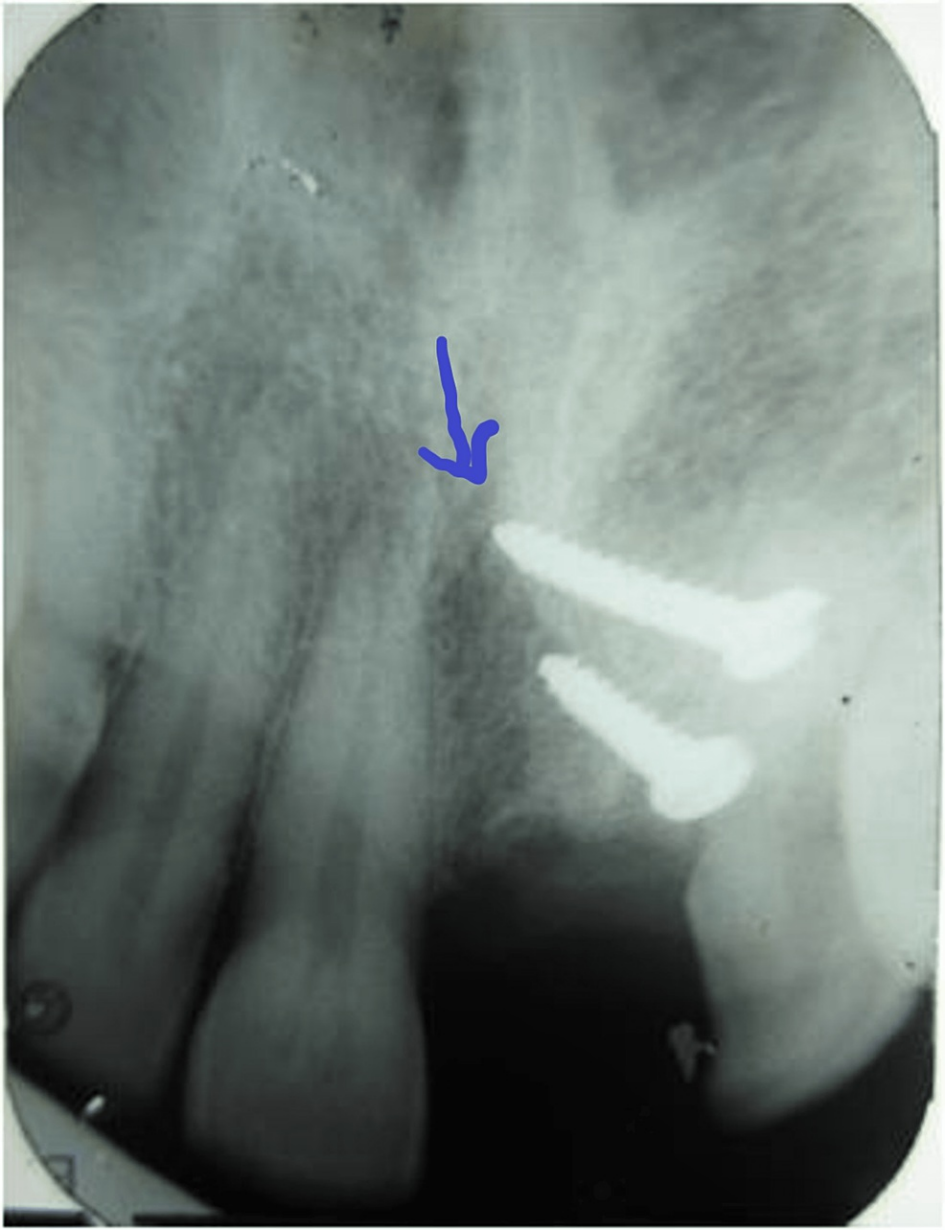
Intraoral periapical radiographs showing fixation screws
